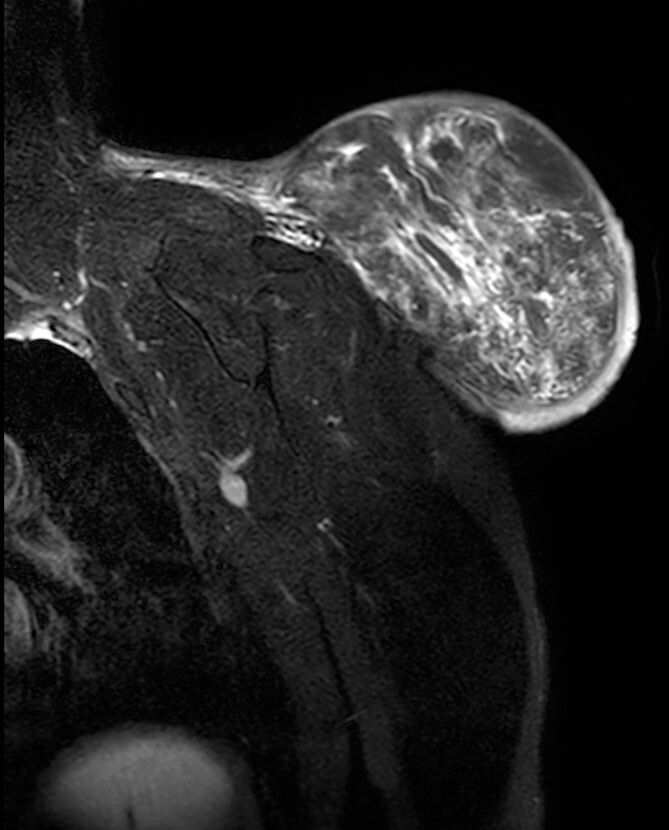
Magnetic resonance imaging T2 with contrast: giant excrescent lesion of 19 × 18 × 14.4 cm of diameter located in the subcutaneous tissue of the posterior aspect of the scapula divided by multiple septums; axillary lymph node of ~0.9 cm; images compatible with liposarcoma with a metastatic axillary lymph node.
